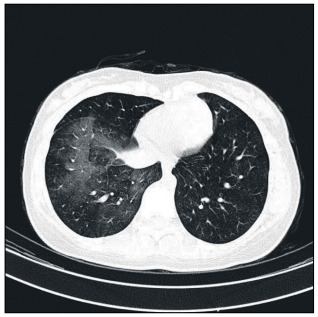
Computed tomography scan of the chest (pulmonary window), depicting patchy alveolar hemorrhage in right lower lobe.
